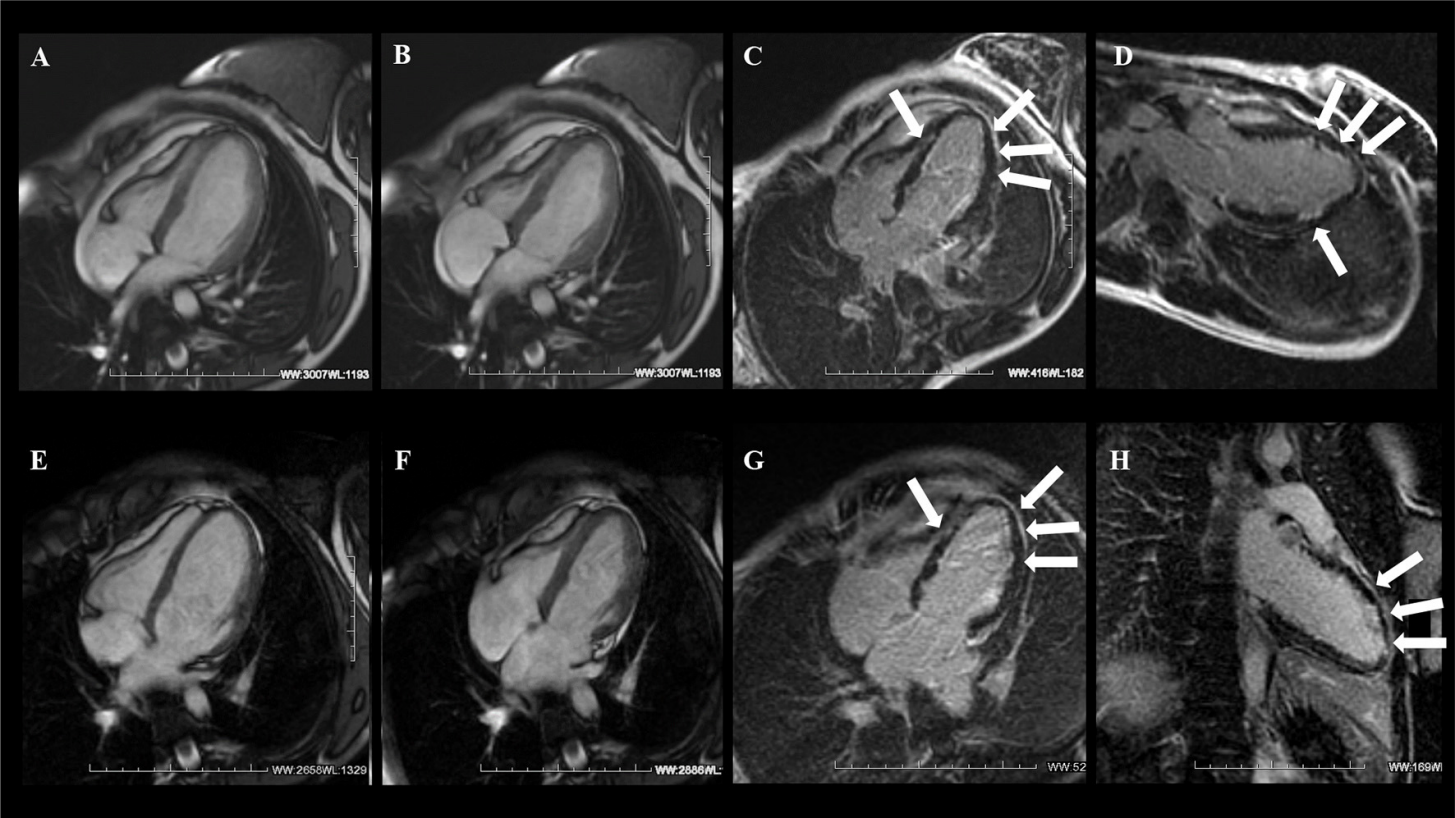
A 21-year-old woman with sudden onset of dyspnea 1 week after delivery and without prior medical history. CMR examination with cine balanced steady-state free precession (bSSFP) sequence four-chamber view [end-dyastole (A) and end-systole (B)] showed severely reduced LV systolic function without RV involvement. (C,D) T1-weighted inversion recovery gradient echo (T1w-IR-GRE) sequence acquired 10 min after gadolinium administration showed diffuse subendocardial late gadolinium enhancement (LGE) of the LV (arrows). At 6 months' follow-up, there was evidence of LV functional recovery (E,F) with diffuse LGE persistence (G,H). Courtesy of Dr. Giovanni Donato Aquaro, Fondazione Toscana Gabriele Monasterio, Pisa, Italy.
